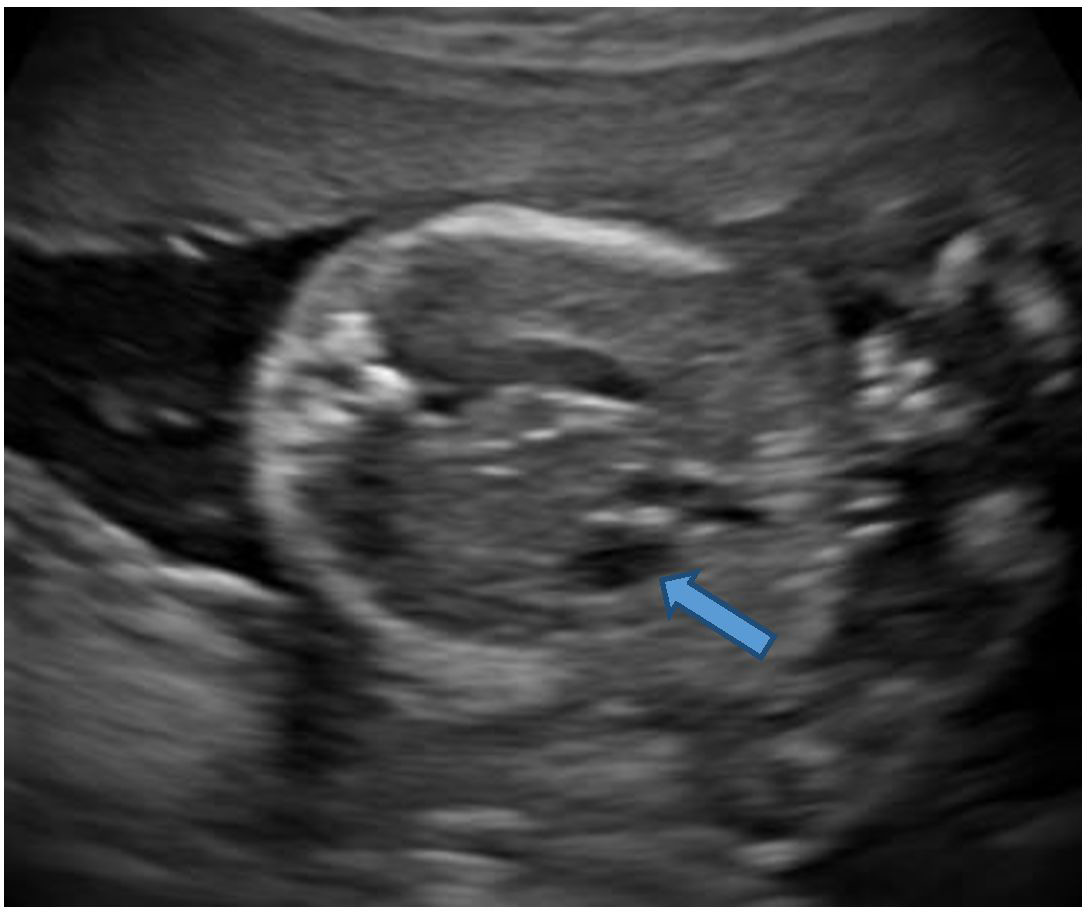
Ultrasound. Axial section of the upper abdomen shows a small hepatic cyst (block arrow).
